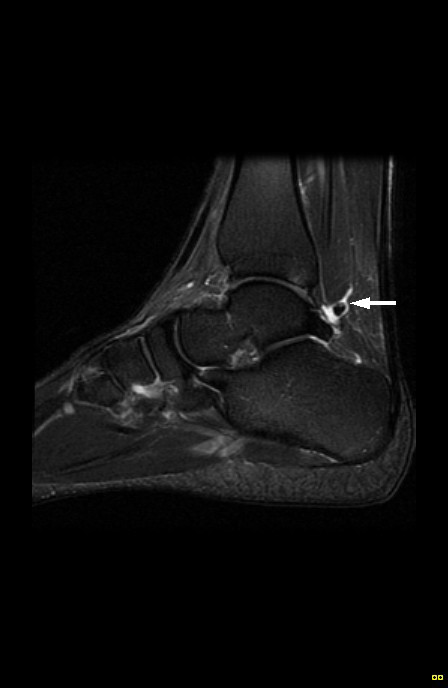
Sagittal MRI of the right ankle (fat-saturated T2-weighted) revealing a predominantly low signal intensity nodule in the synovial sheath of the flexor hallucis longus tendon (arrow).
